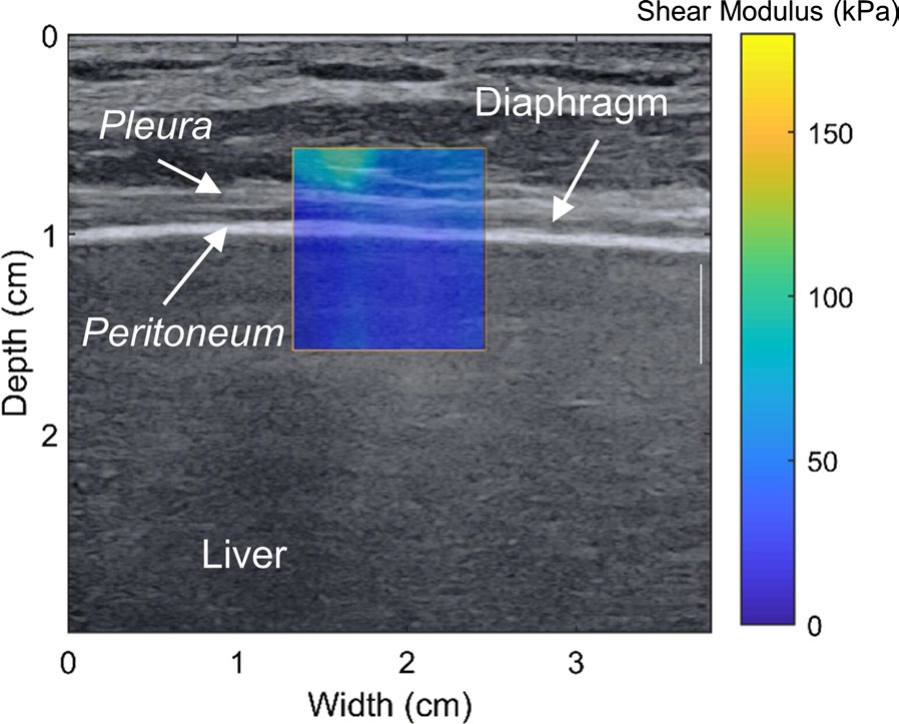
Typical ultrasound image obtained during shear wave elastography imaging of the diaphragm. Shear modulus map obtained from ultrasound shear wave elastography overlaid with standard ultrasound B-Mode during intercostal scanning of the diaphragm at the right zone of apposition
